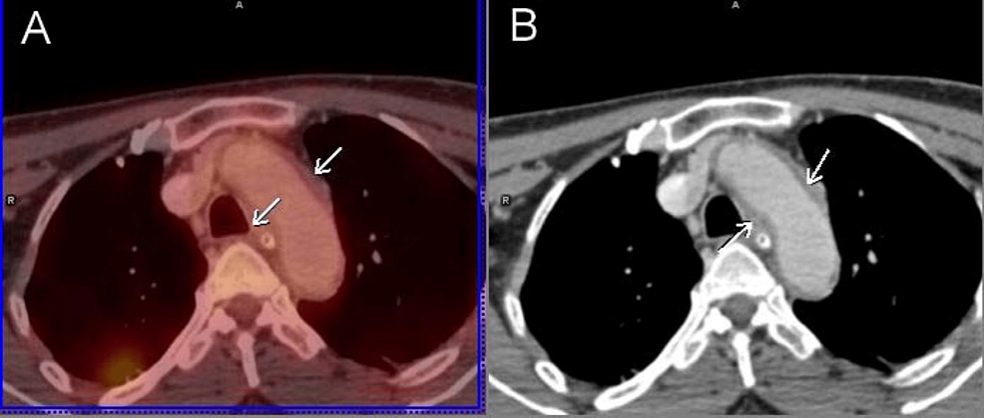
(A) Positron emission tomography/computed tomography (PET/CT) image showing thickening of the vessel wall of the aortic arch with diffuse low-grade uptake (white arrows). (B) Corresponding CT image showing vessel wall thickening (white arrows).
